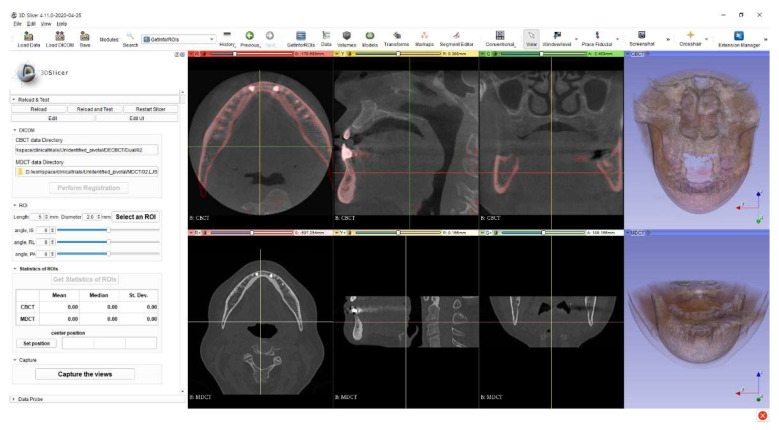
A screenshot of the developed 3D slicer module. Registered multi-detector computed tomography (MDCT) volume was represented as the red shade upon dual energy (DE) cone beam computed tomography (CBCT) volume.
